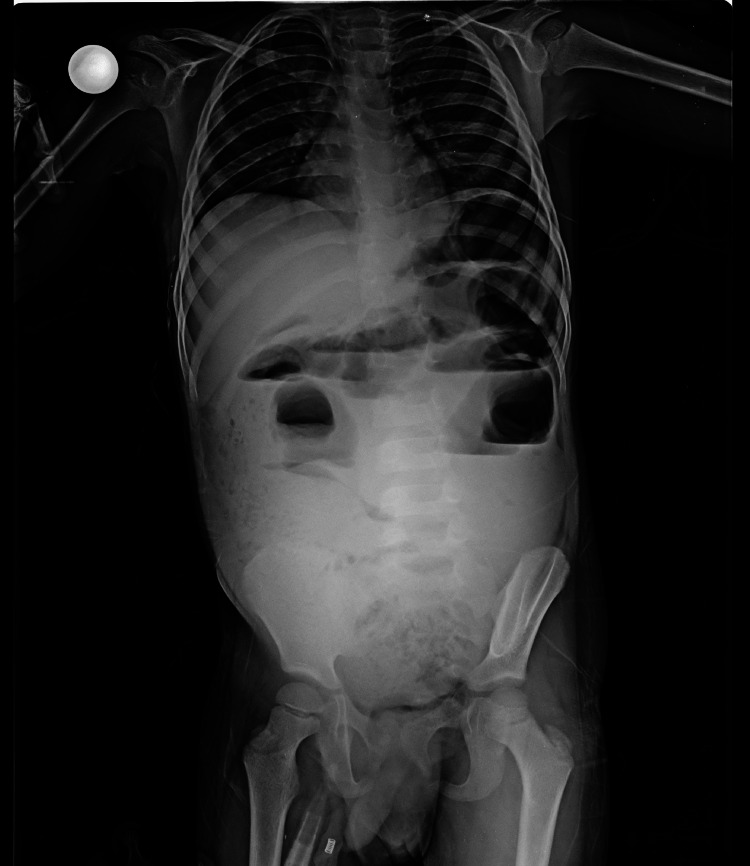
The X-ray of the abdomen showing multiple air-fluid levels
